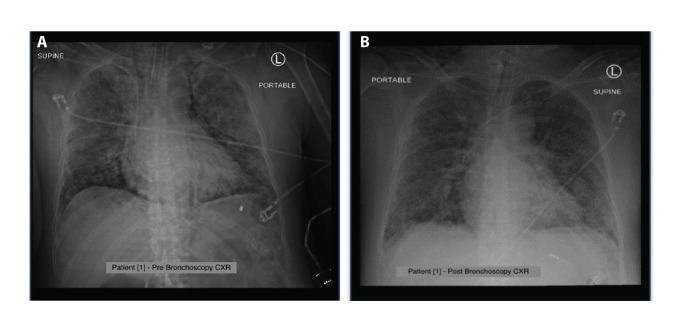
Chest radiograph for patient 1 (A) pre- and (B) post-bronchoscopy
